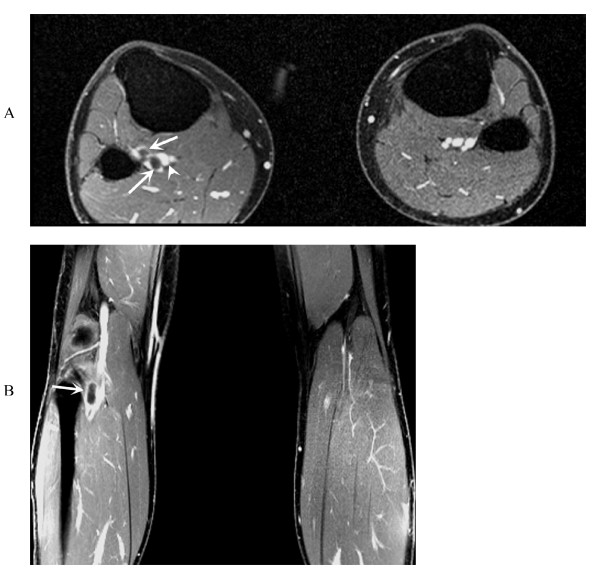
Axial and coronal T1-weighted post-contrast fat saturated gradient echo (GRE) images show no evidence of contrast enhancement of the well defined hypo-intense cystic mass. Compression of the popliteal arterial lumen (figure 2a and 2b, arrows), and normal enhancement of the popliteal vein (figure 2a, arrowhead) are noted.
